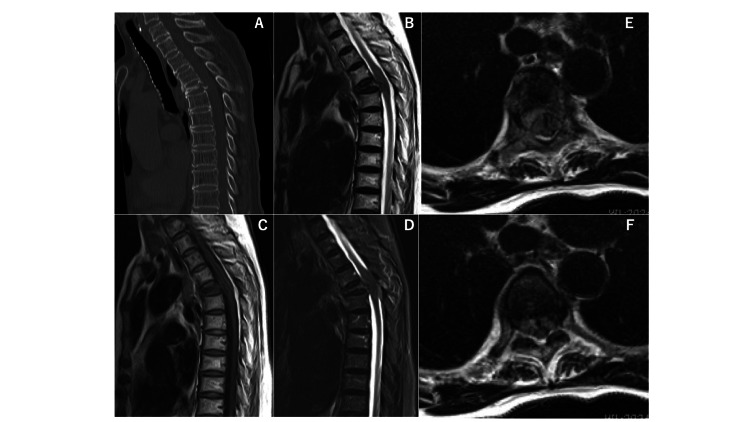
Computed tomography image and magnetic resonance images on arrival Computed tomography image (CT) shows a Th5 level vertebral fracture (A). Magnetic resonance images show the Th5 vertebral fracture and spinal compression at the same level (B: T2-weighted image, C: T1-weighted image, D: STIR. MRI T2-weighted axial images show spinal compression from the ventral side continuous vertebral body (E, F).
